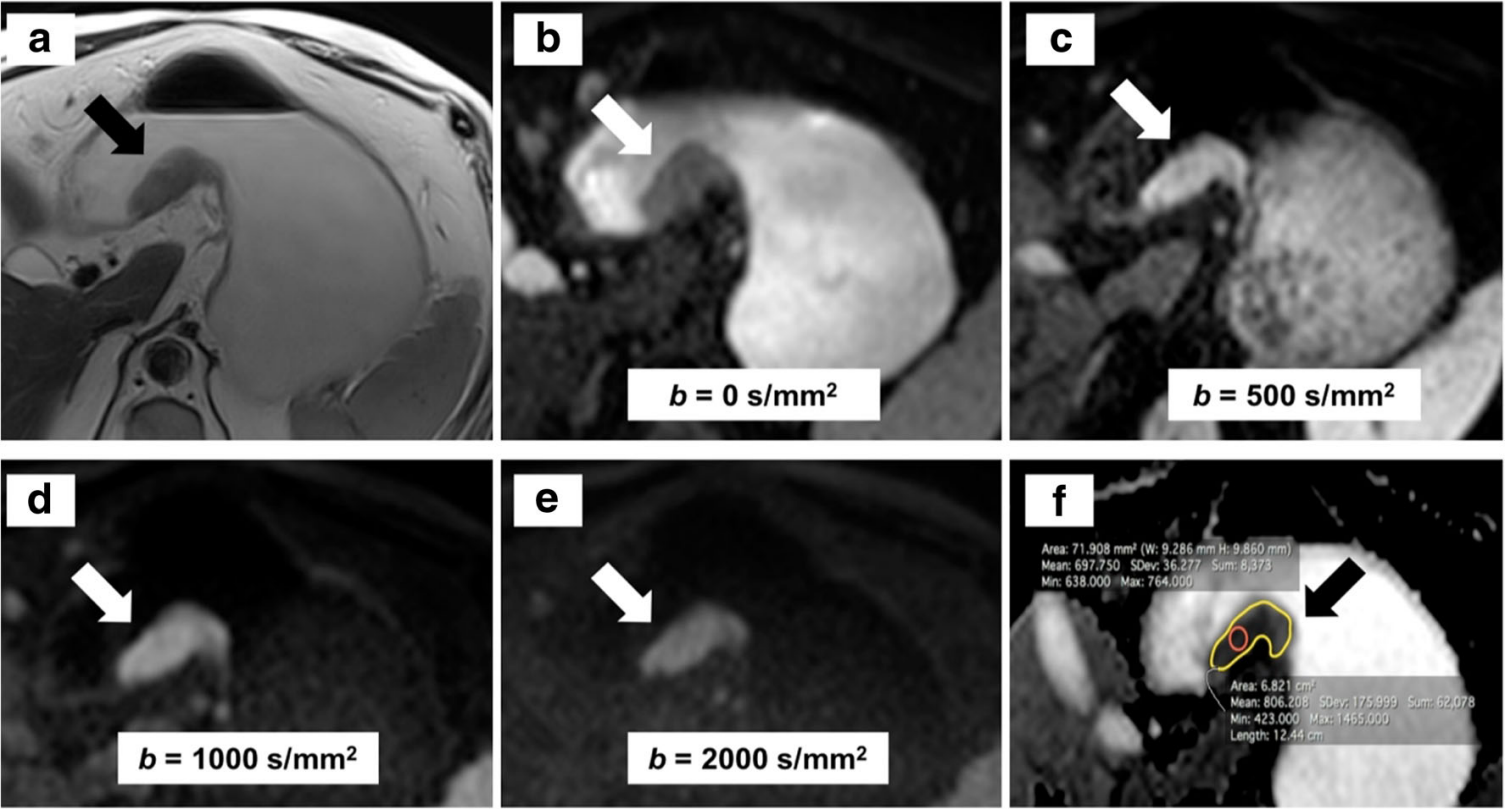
The arrows indicate a tumour of the lesser curvature on T2-weighted imaging (a) and on diffusion-weighted magnetic resonance imaging (DW-MRI) using different *b* values (b–e). The apparent diffusion coefficient (ADC) is calculated on the ADC map (f) from a small (red) or large (yellow) region of interest (red) on a single slice
